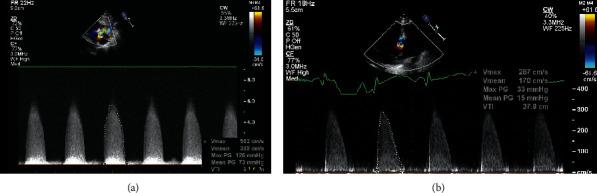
(a) An example of echocardiography preballoon angioplasty, continuous-wave spectral Doppler across the aortic valve in a newborn showing severe aortic stenosis with a mean gradient 73 mmHg and a peak systolic gradient 126 mmHg. (b). Echocardiography postballoon angioplasty, continuous-wave spectral Doppler across the aortic valve revealing marked decrease in the degree of aortic stenosis to a mean gradient of 15 mm Hg and a peak systolic gradient of 33 mmHg.
